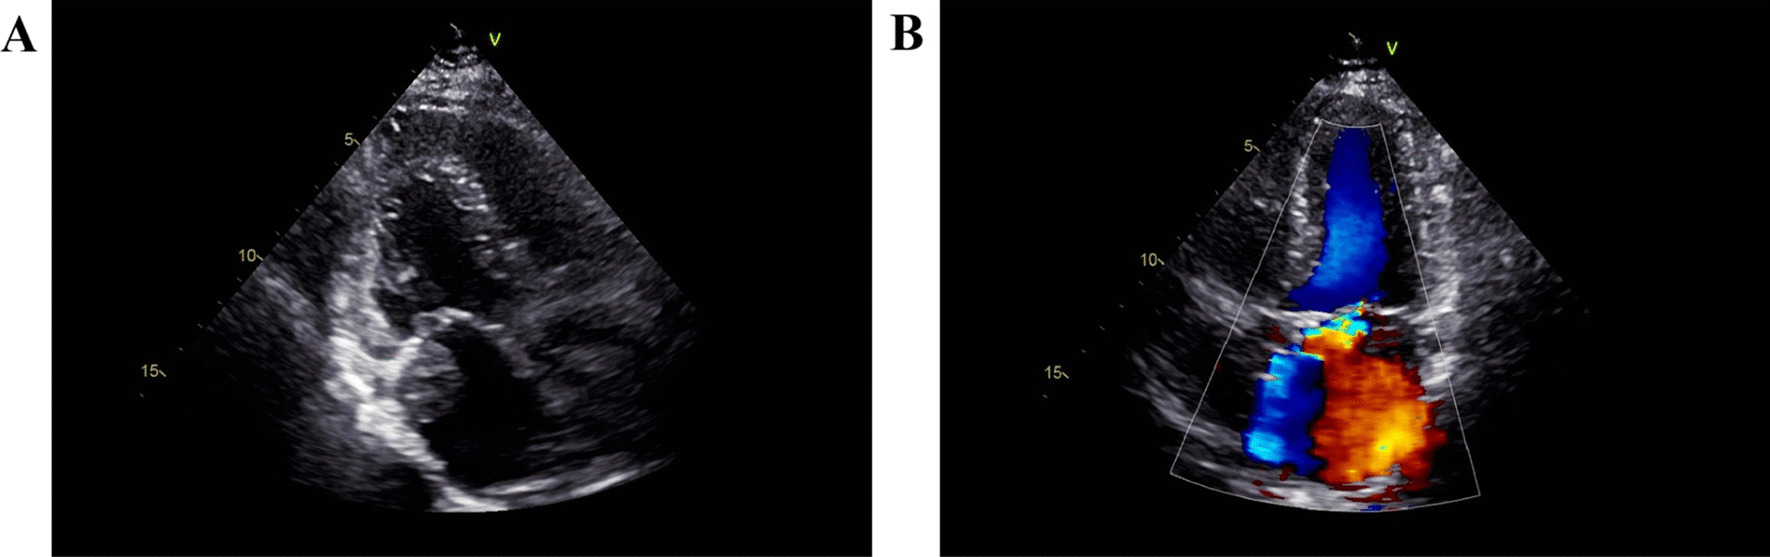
Postoperative ultrasound image of myxofibrosarcoma in the left atrium. (A) Two-dimensional ultrasound image of myxofibrosarcoma. (B) Ultrasound image of diastolic mitral flow in myxofibrosarcoma
